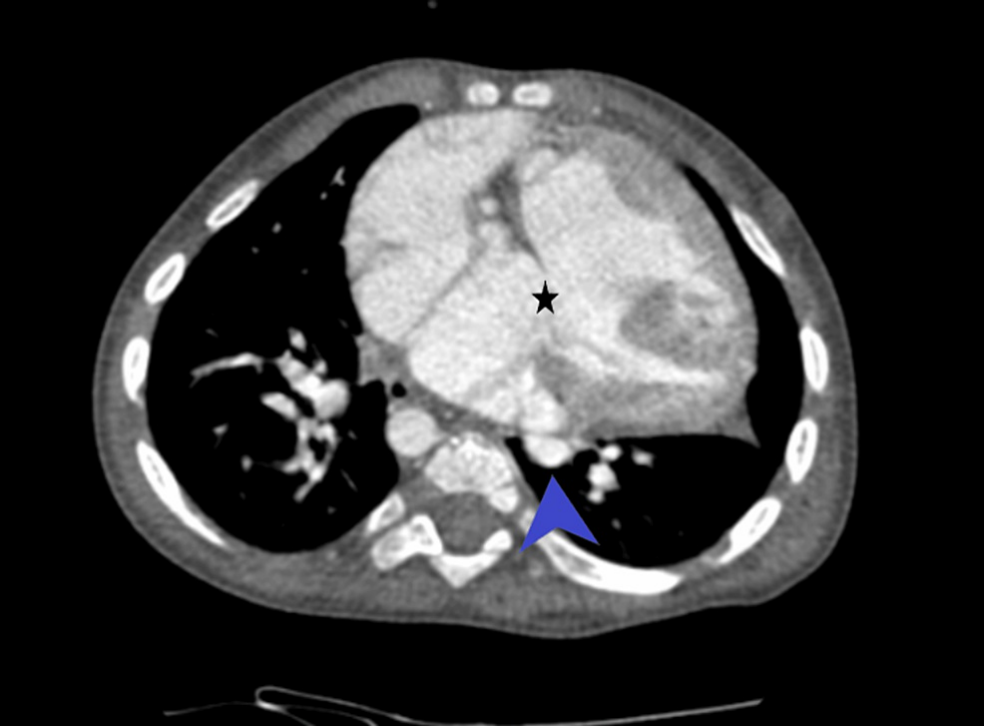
Computed tomography pulmonary angiogram revealing multiple direct and indirect major aortopulmonary collaterals supplying the pulmonary arteries (blue arrowhead), large atrial septal defect, and ventricular septal defect (asterisk).
